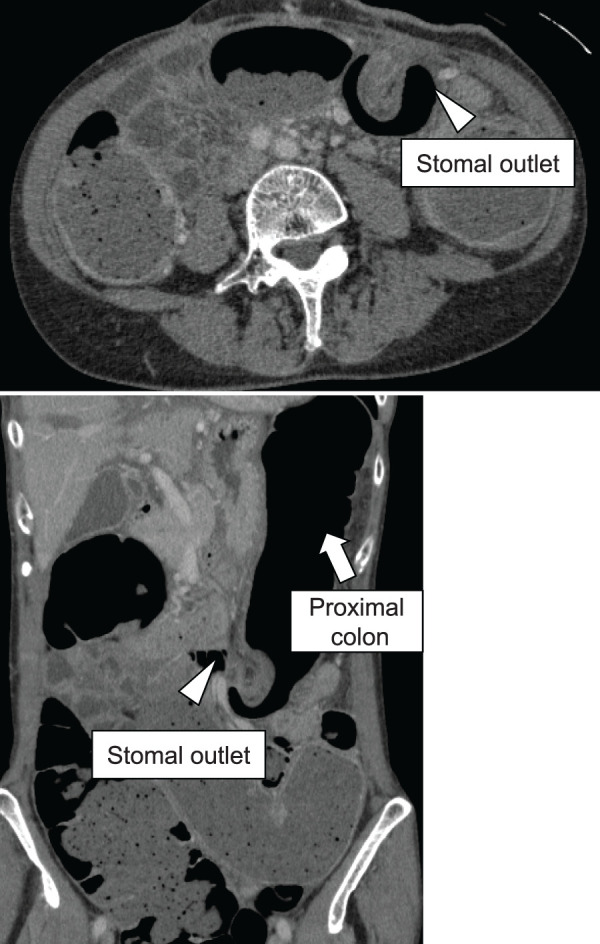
Contrast-enhanced CT showing stenosis at the stomal outlet (arrowhead) with marked dilatation of the proximal colon (arrow).
